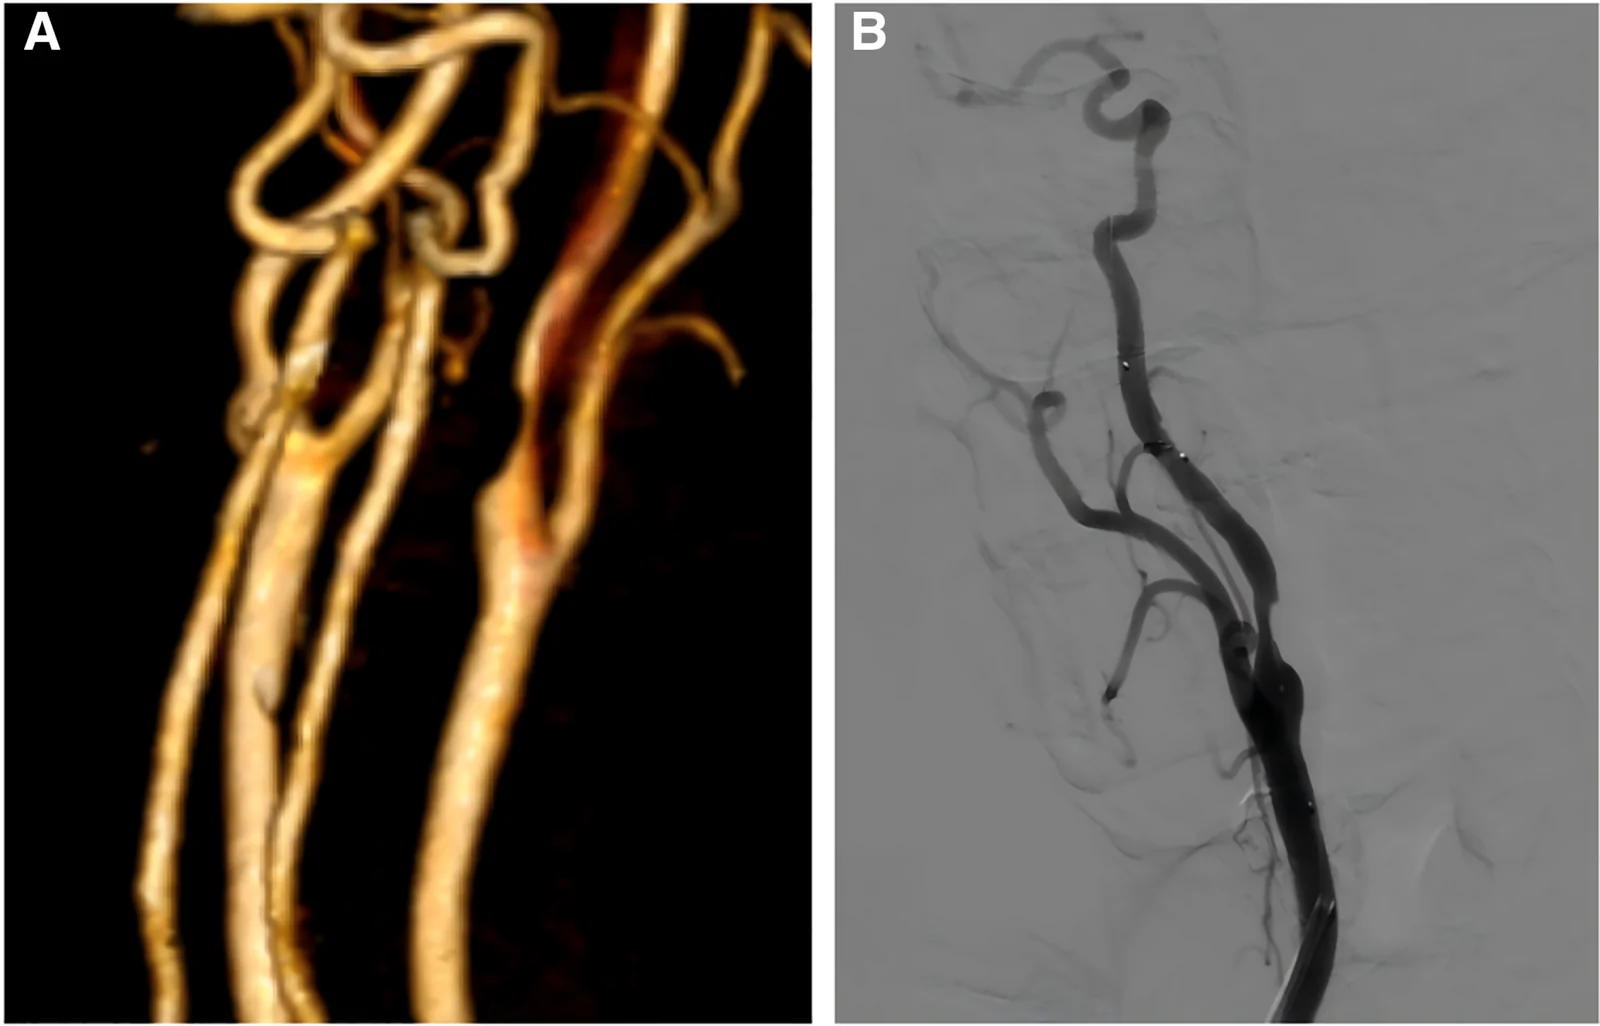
Head and neck CTA and cerebral angiography revealed stenosis of the right internal carotid artery. CTA = computed tomography angiography.
